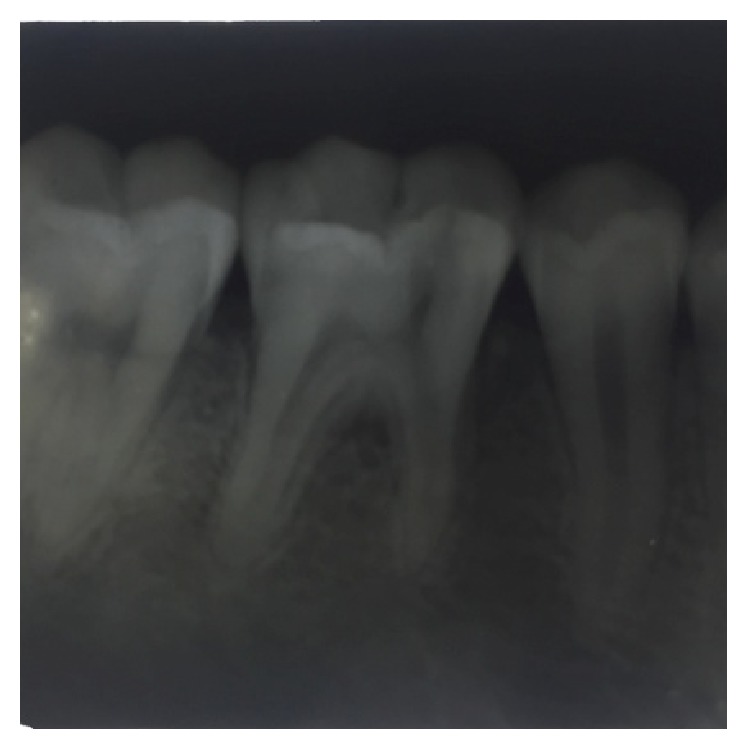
Preoperative radiograph of 46.
